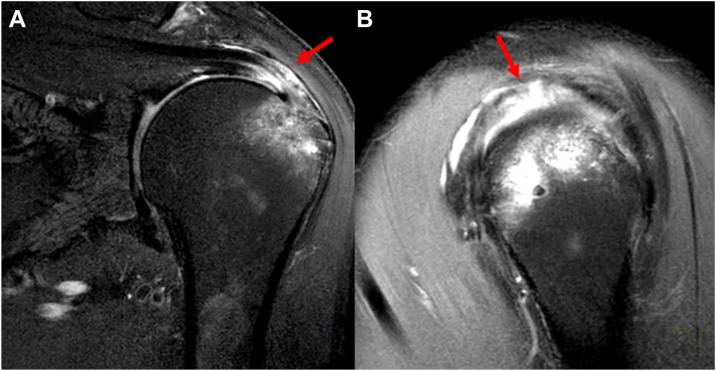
Preoperative magnetic resonance imaging (MRI) identifying a high-grade partial articular-sided supraspinatus retear (PASR). (A) A coronal T2-weighted fat-suppressed image reveals the PASR lesion. The *red arrow* indicates abnormal high signal intensity along the articular side of the supraspinatus tendon, while the bursal side remains intact. (B) A sagittal oblique view confirms the extent of the articular-sided defect (*red arrow*), clearly distinguishing it from a full-thickness tear. These multiplanar images provided a precise assessment of the high-grade retear.
